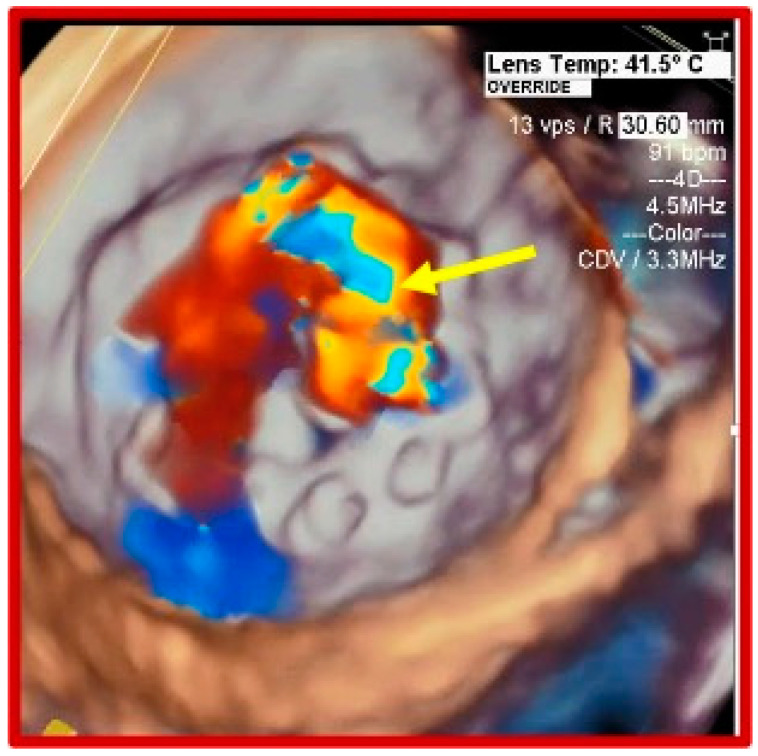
Three-dimensional color doppler transesophageal ECHO showing a large mitral regurgitant get.
